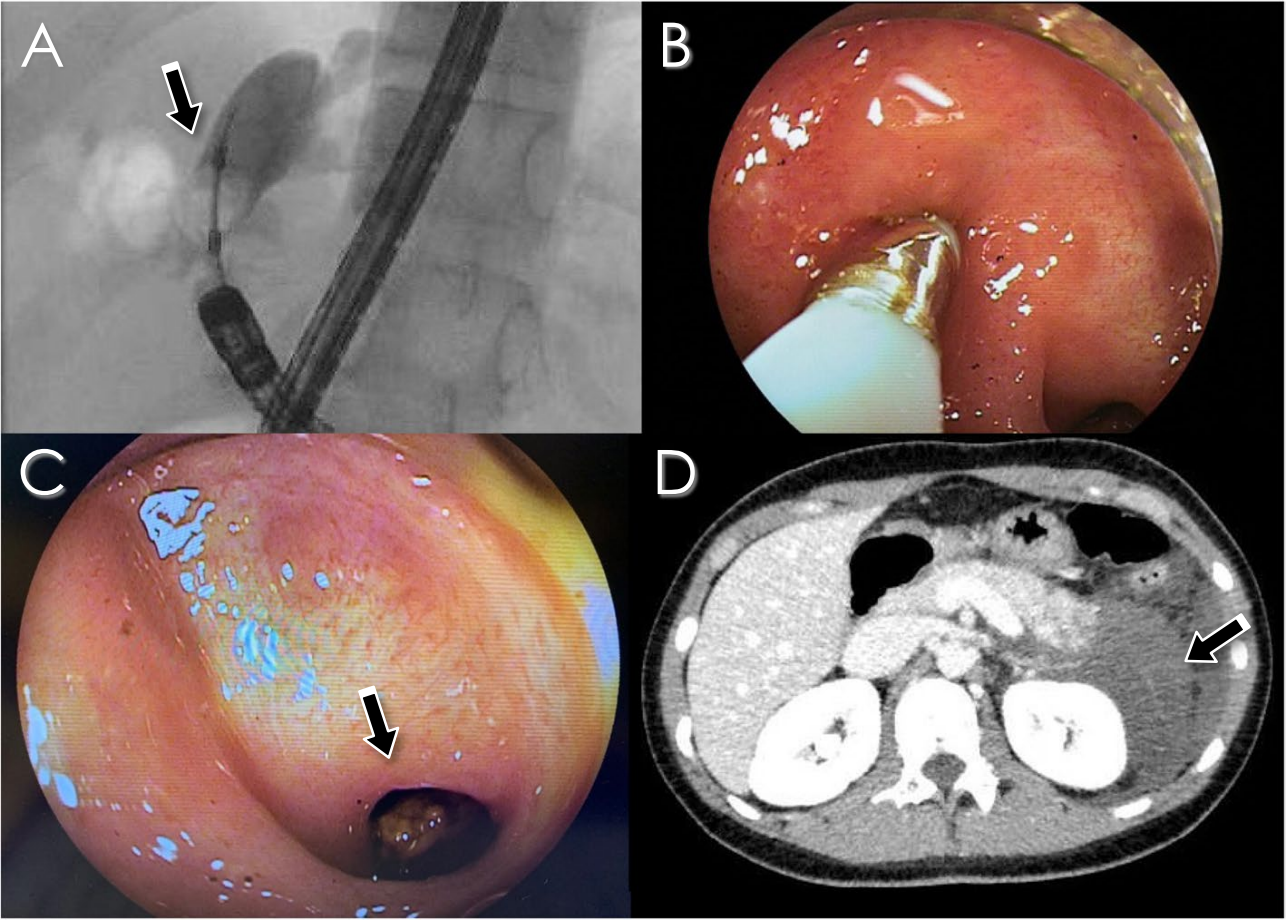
DB-ERC findings and acute pancreatitis after intervention (Case 3). A Cholangiography and balloon dilatation of right hepatic duct stricture. B In DB enteroscopy, the stone was crushed using a crusher catheter. C Arrow shows the IHBD stone. D Enhanced abdominal CT shows decreased enhancement and swelling of the pancreatic tail 1 day after DB-ERC
